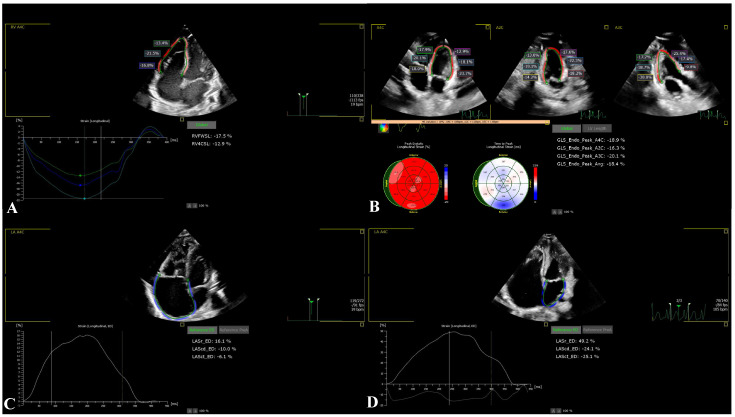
Representative speckle-tracking echocardiography (STE) analysis in a neonate with Ebstein’s anomaly. Legend: (A) Right ventricular longitudinal strain analysis from the apical four-chamber view, including free-wall and four-chamber strain curves; (B) Left ventricular global longitudinal strain (LV_GLS) derived from apical four-, two-, and three-chamber views, with corresponding bull’s-eye plot; (C) Left atrial strain analysis demonstrating reservoir, conduit, and contractile phases; (D) Right atrial strain analysis demonstrating impaired atrial deformation.
